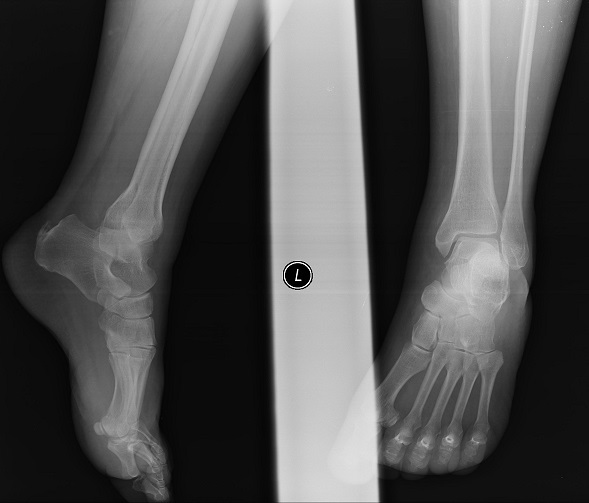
On direct x-ray examination in Emergency room. Anteroposterior (a) and lateral X-ray of the ankle
